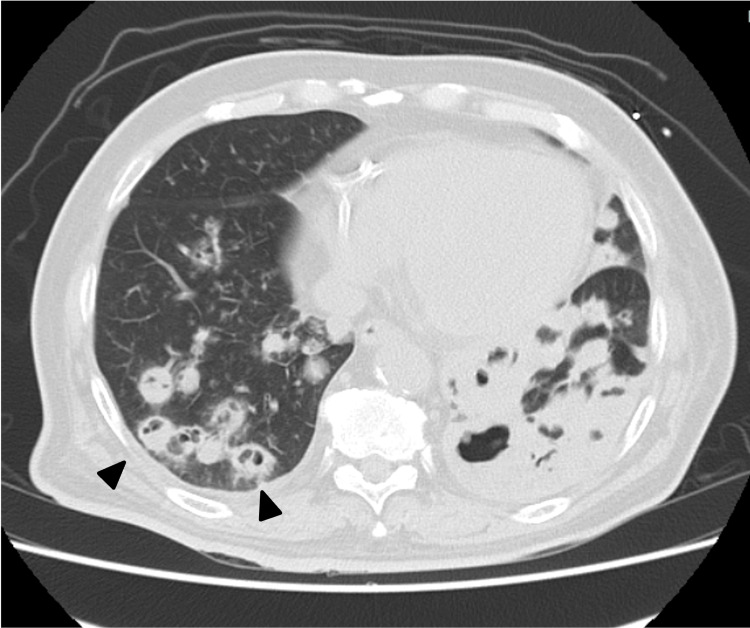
Chest computed tomography on Day X+7 displaying the development of multiple cavitary lesions in the previously infiltrated areas.
